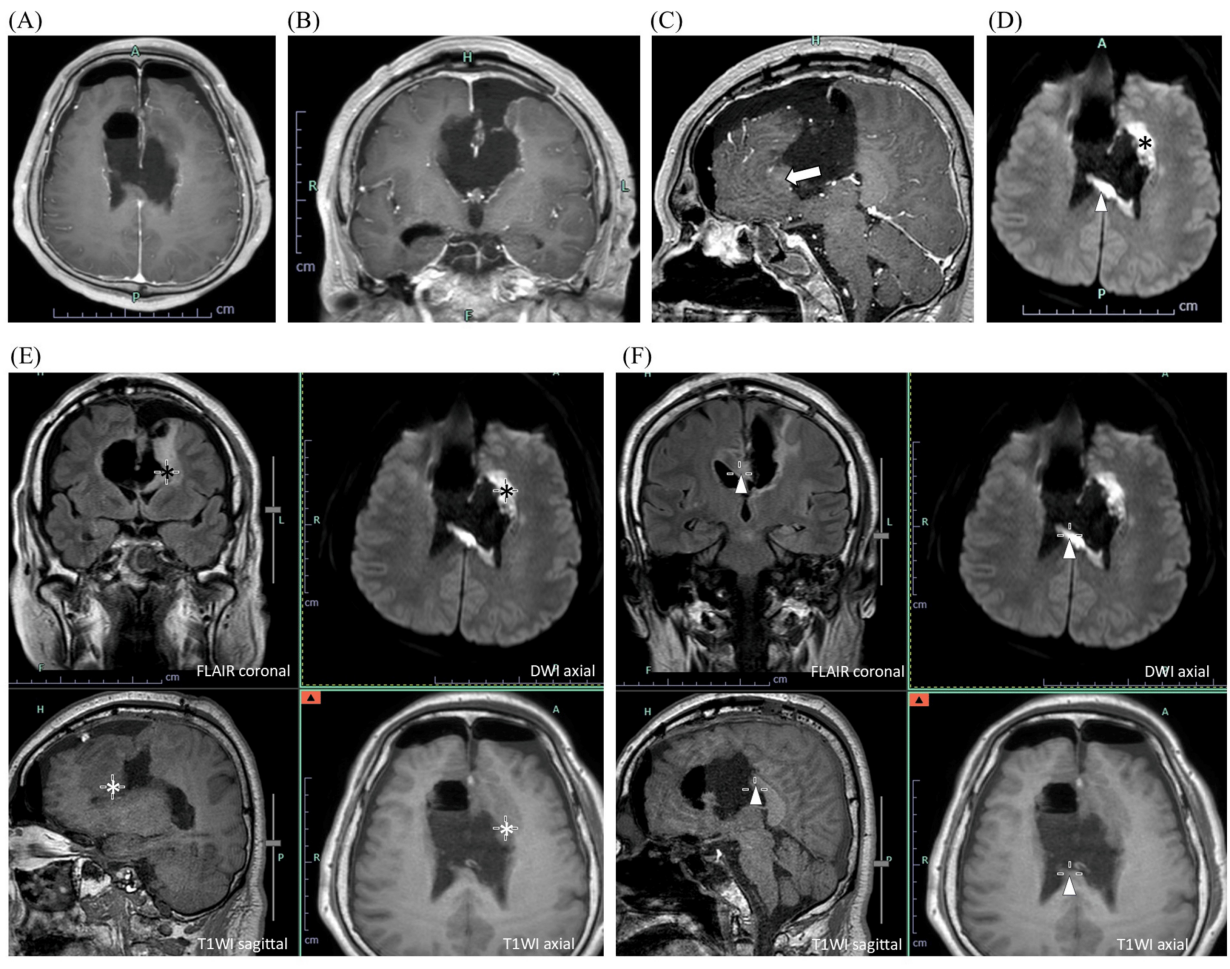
Postoperative MRI findings (second surgery). Postoperative brain MRI after the second surgery demonstrates near-total resection of the enhancing lesions on post-contrast T1-weighted images (T1WIs) in axial (A), coronal (B), and sagittal (C) sequences. The genu of the corpus callosum appears structurally intact (blank arrow); however, DWI reveals cerebral infarction involving the genu (asterisks) and the posterior body (arrowheads) of the corpus callosum on diffusion-weighted images (D-F). The same regions are also shown in fused images combining FLAIR, T1WI, and DWI sequences. DWI: Diffusion-weighted image; FLAIR: Fluid-attenuated inversion recovery; WI: Weighted image.
